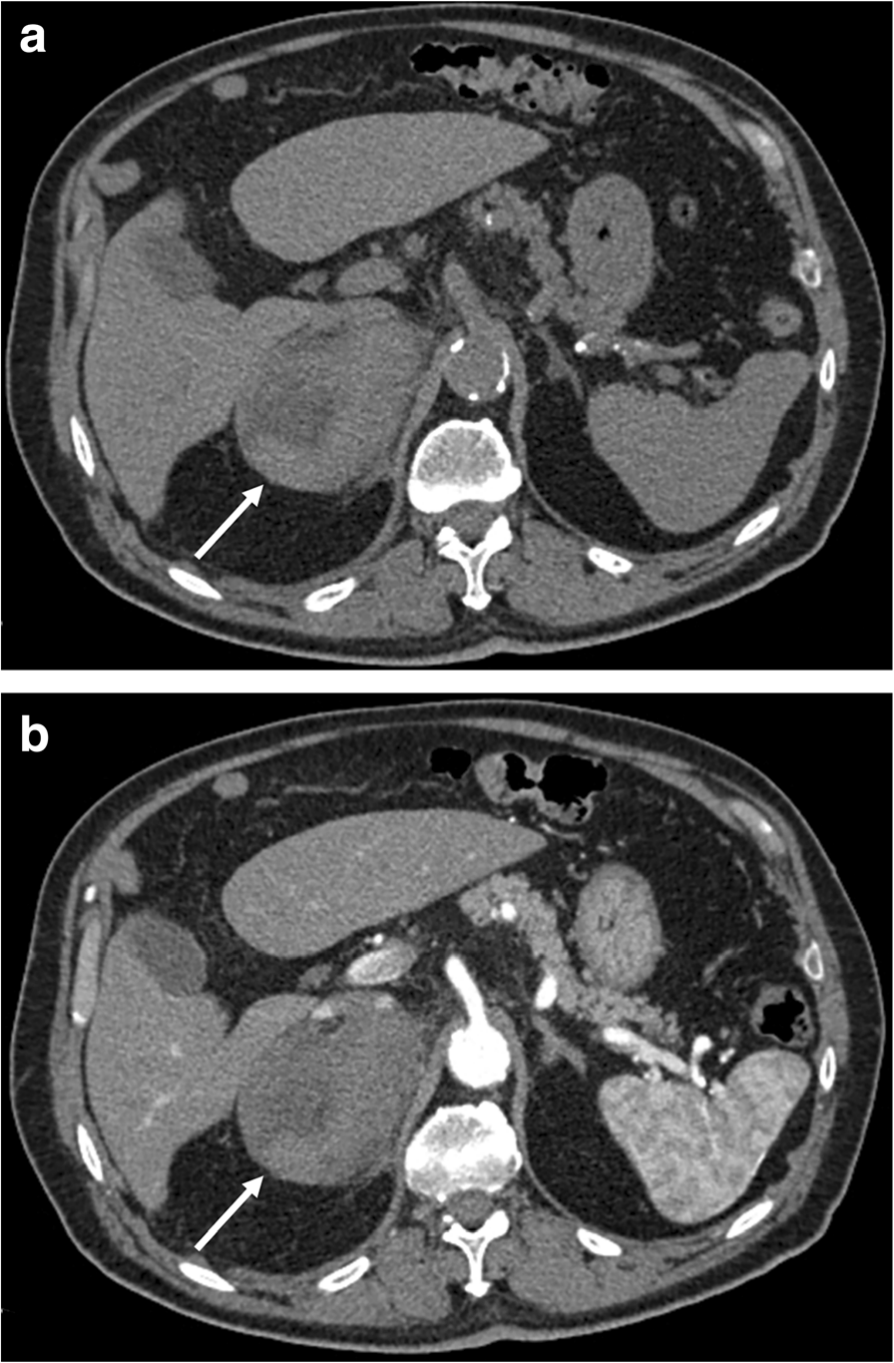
Right adrenal hemorrhage in a 61-year-old man with acute abdominal pain after starting anticoagulant therapy. Axial unenhanced (a) and arterial (b) phase CT images show a large right adrenal hematoma (arrow) with relatively high attenuation values and without enhancement after contrast injection
